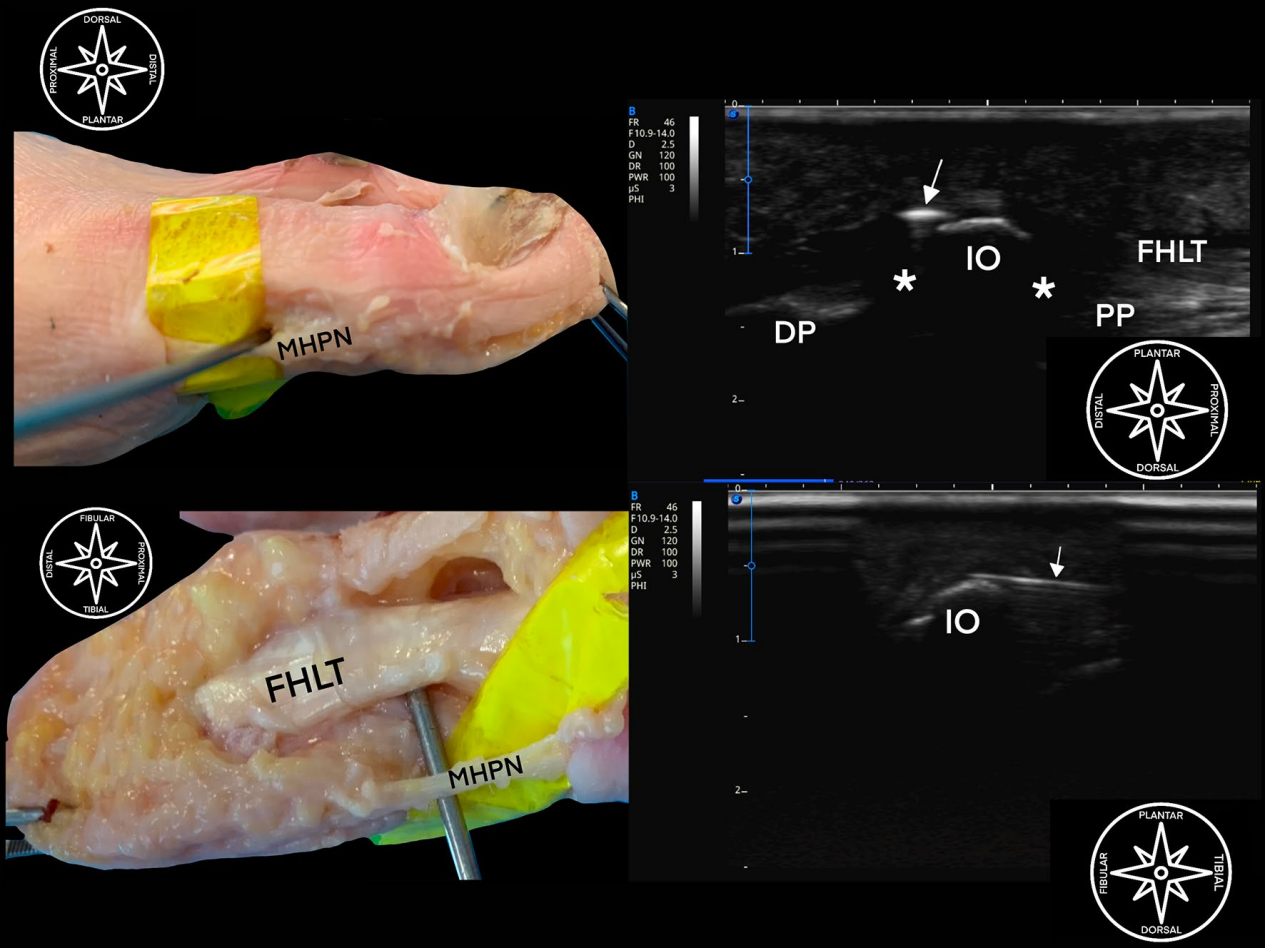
Ultrasound guided shaving of the interphalangeal joint ossicle of a left foot. (A) Dissection representation (tibial aspect) showing minimally invasive burr just dorsal to MHPN (medial plantar nerve). (B) Ultrasound guided shaving; *PP* proximal phalanx, *DP* distal phalanx, *IO* interphalangeal ossicle, *asterisk* * plantar plate, FHLT flexor hallucis longus tendon, white arrow showing burr (out of plane) between FHL and IO. (C) Dissection representation (plantar aspect) showing minimally invasive burr just dorsal to MHPN (medial plantar nerve); FHLT flexor hallucis longus tendon. (D) Ultrasound guided shaving; *IO* interphalangeal ossicle, white arrow showing burr (in-plane) between FHLT and IO.
